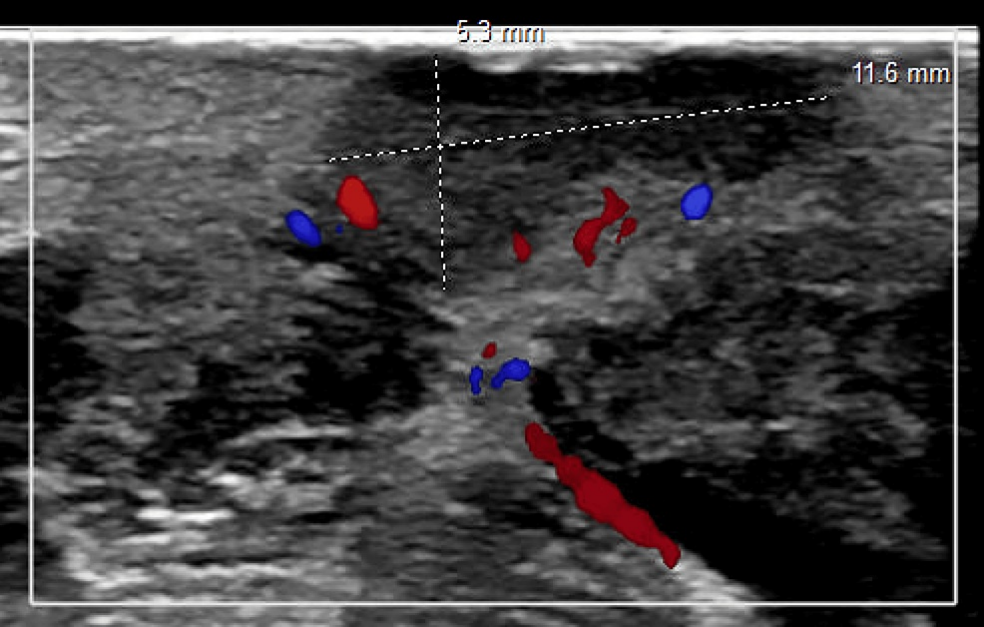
Ultrasound Demonstrating Fluid Collection at Site of Surgery
